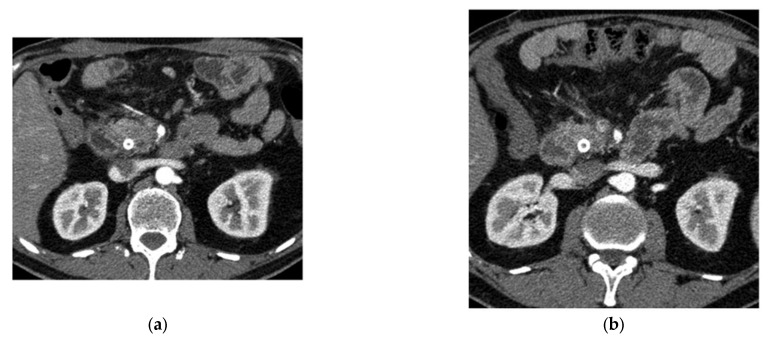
CT study of pancreatic adenocarcinoma before and after chemotherapy: (a) CT study of locally advanced ductal adenocarcinoma of the pancreatic head; (b) CT control after chemotherapy [118].
